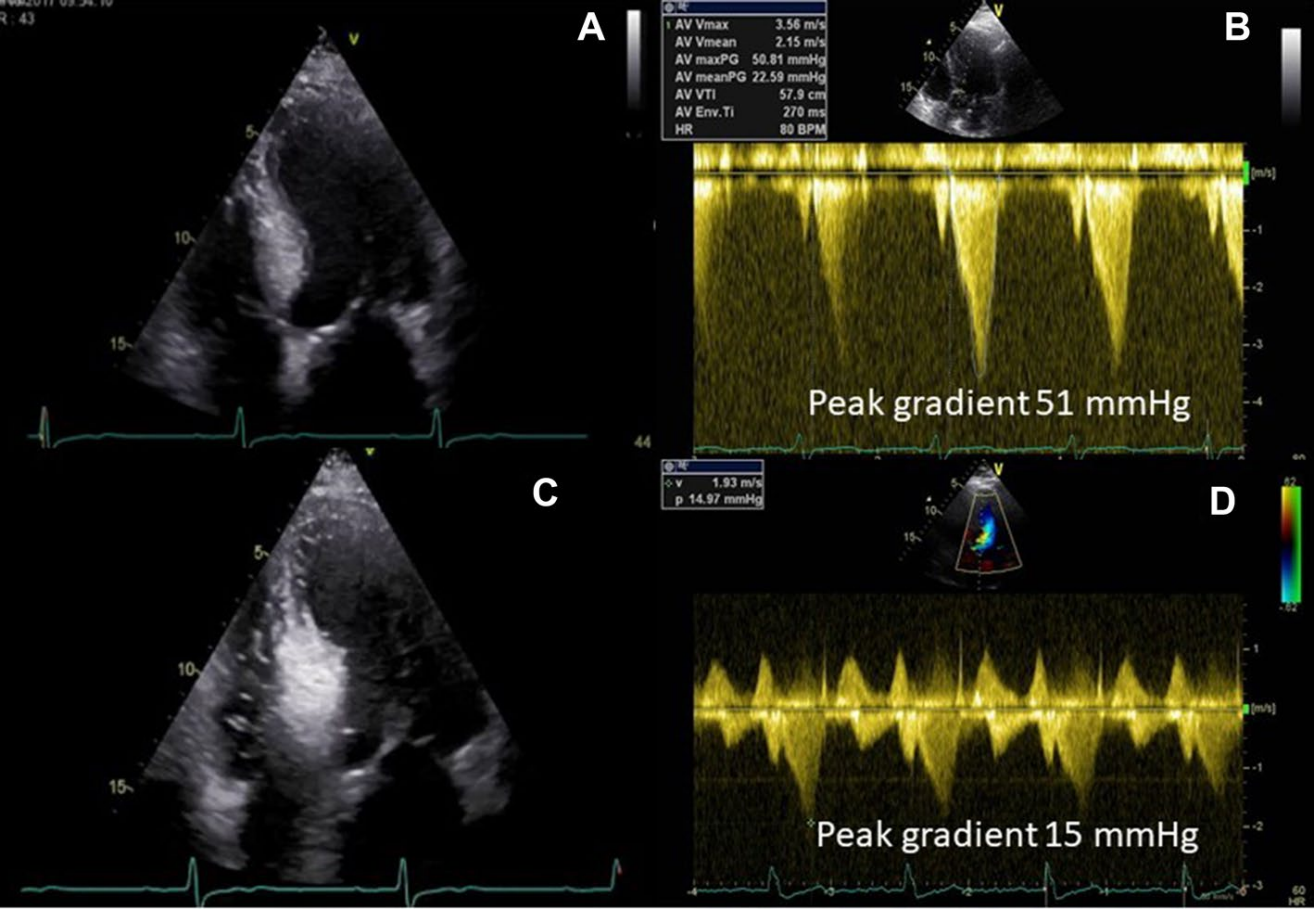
Patient with septal hypertrophy and MWT of 23 mm (a) with peak gradient of 51 mmHg at rest (b). Echocardiography with injection of contrast in septal branch of the coronary artery with supply of the basal part of the septum (c). Peak gradient of 15 mmHg after ASA (d)
